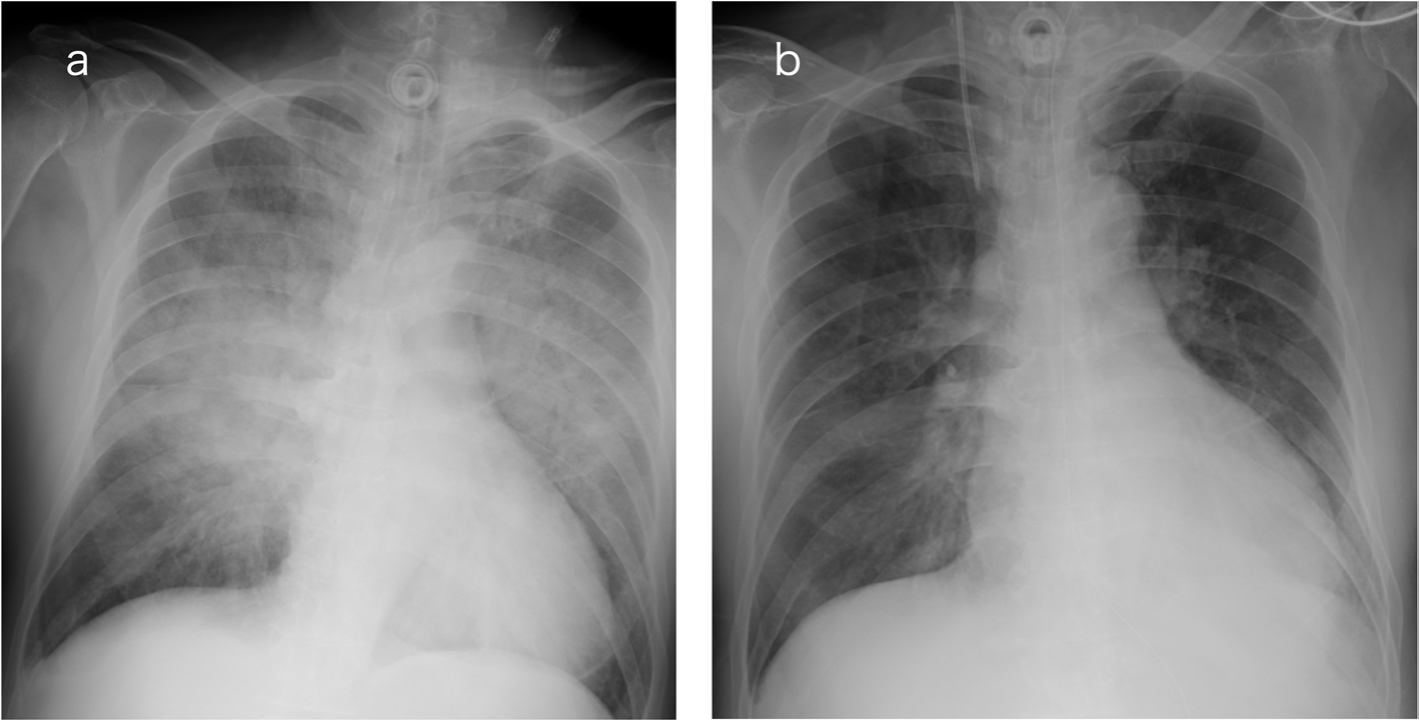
Chest radiography images of Case 1. a At the onset of pulmonary edema after tracheostomy. b Forty-eight hours after onset and treatment of pulmonary edema
